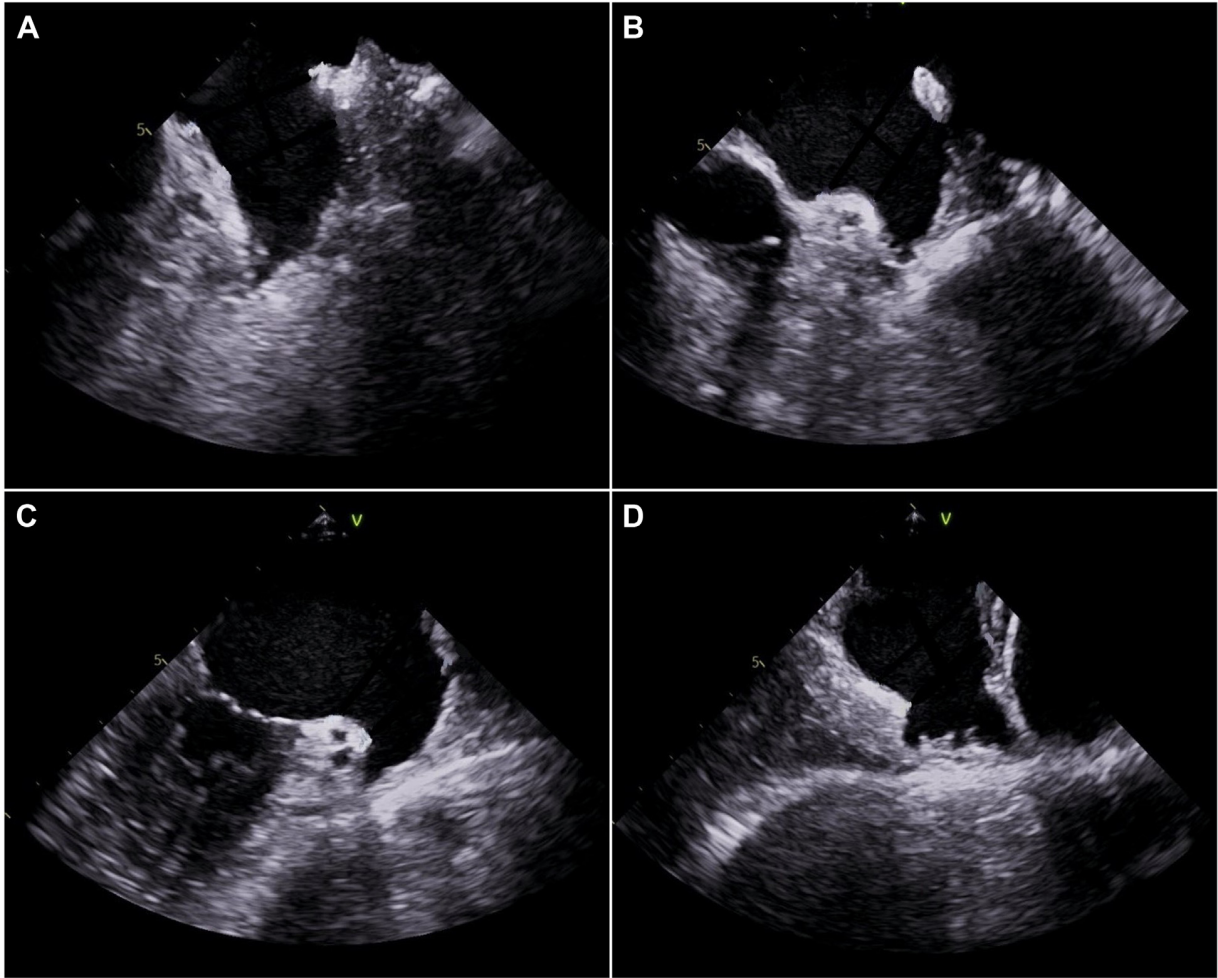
LAA Transesophageal Echocardiogram The left atrial appendage was visualized in the 0° (A), 45° (B), 90° (C), and 135° (D) views. The maximal left atrial appendage (LAA) ostium width and depth were 24 mm and 12 mm, respectively.
